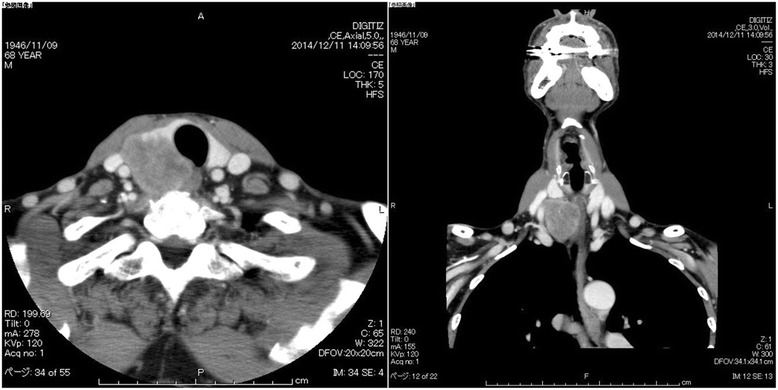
CT findings. A low-density mass of approximately 5.0 cm in the right lobe of the thyroid-induced tracheal and esophageal deviation and deformation
